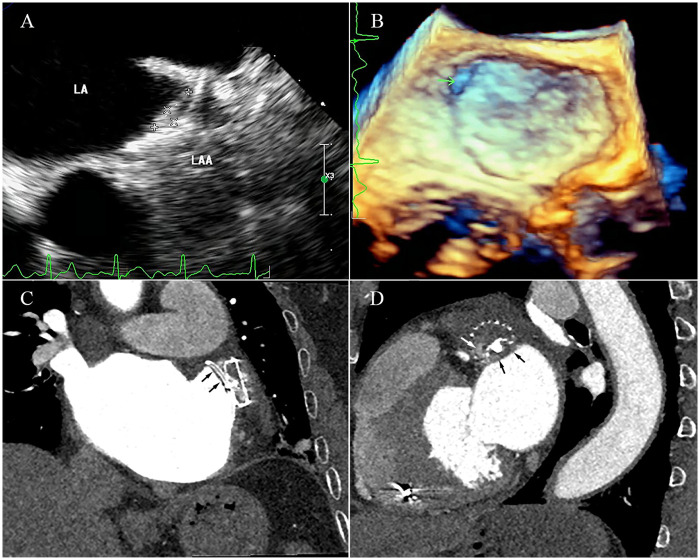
Multimodality imaging appearances of device-related thrombus and hypoattenuated thickening after left atrial appendage occlusion. (A) Two-dimensional transesophageal echocardiography demonstrates a mass-like echogenic lesion attached to the atrial surface of the occluder device; caliper markers delineate the lesion extent, consistent with device-related thrombus. (B) Three-dimensional transesophageal echocardiography further depicts the spatial relationship between the surface-attached lesion and the occluder device (green arrow). (C) Coronal cardiac computed tomography angiography shows a thin, smooth, mural hypoattenuated layer along the device surface (black arrows), consistent with low-grade hypoattenuated thickening. (D) Sagittal cardiac computed tomography angiography demonstrates a focal, thicker, protruding hypoattenuated lesion on the device surface (black arrows), consistent with high-grade hypoattenuated thickening; the associated peri-device leak is indicated by a white arrow. CCTA, cardiac computed tomography angiography; DRT, device-related thrombus; HAT, hypoattenuated thickening; LA, left atrium; LAA, left atrial appendage; PDL, peri-device leak; TEE, transesophageal echocardiography.
